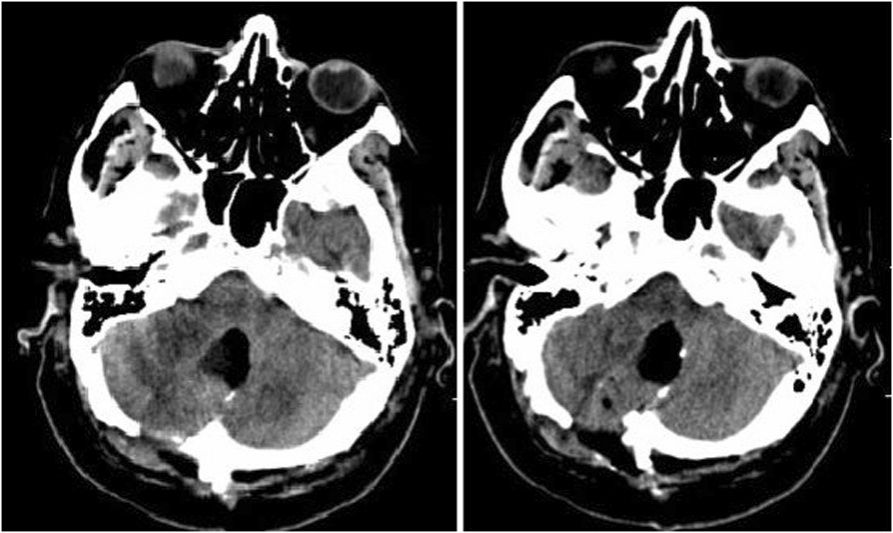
Postoperative computed tomography image demonstrating a partial removal of the lesion
